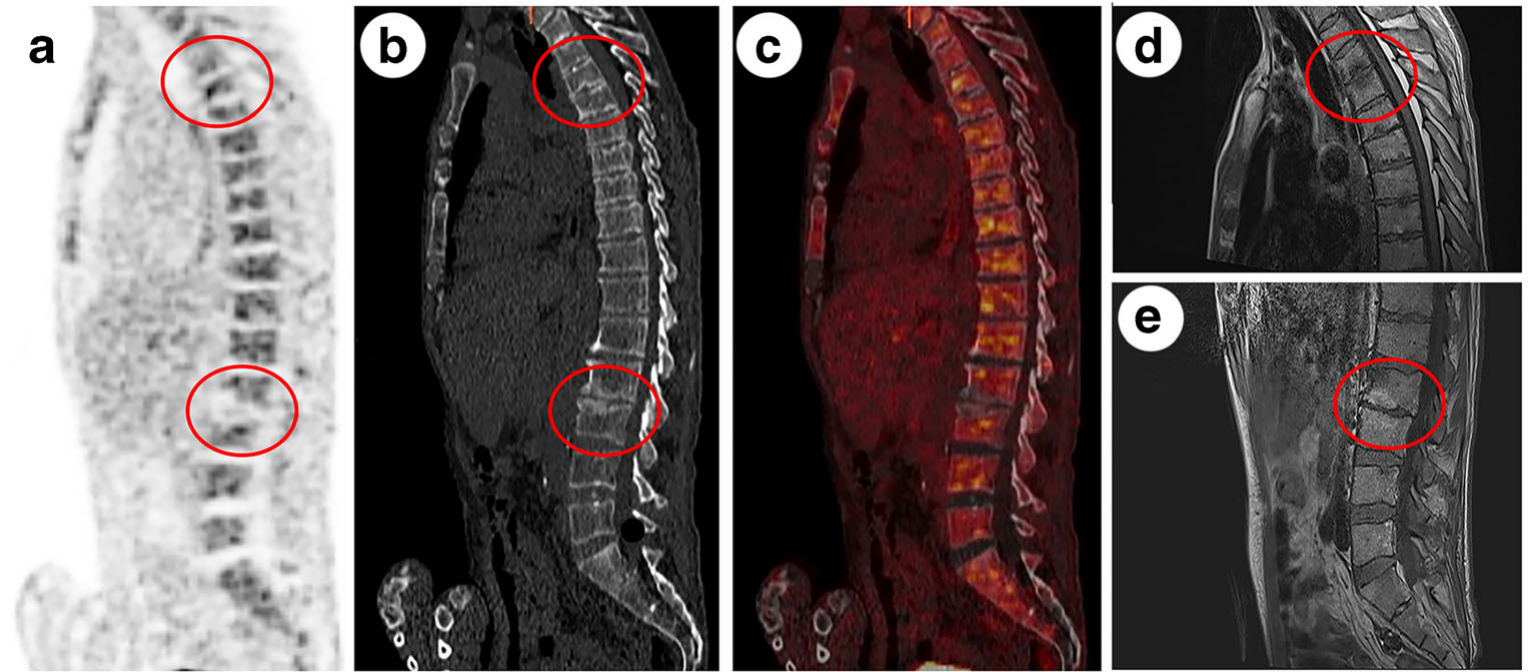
^18^F–FDG-PET/CT and MRI of a 61-year-old man who was admitted with *S. aureus* endocarditis and back pain. ^18^F–FDG-PET (a), CT (b), and ^18^F–FDG-PET/CT (c) showing degenerative changes on level T3-T4 and L2-L3. T1-weighted Gd-chelate enhanced MRI (d, e), pre- and postcontrast, showing Modic type 1 degenerative changes at level T3–4 and L2-L3. In the original MRI report a diagnosis of vertebral osteomyelitis on the levels T3-T4 and L2-L3 was made, ^18^F–FDG-PET/CT was negative for vertebral osteomyelitis. Reevaluation of ^18^F–FDG-PET/CT and MRI were both negative (score 1) and long-term follow-up confirmed that the back pain could be attributed to degenerative changes of the spine
